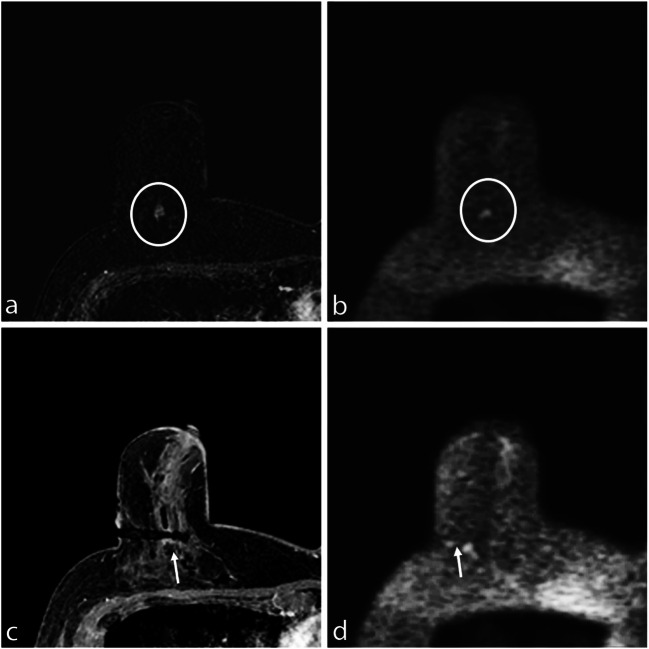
a Subtracted DCE 3D-THRIVE showing a mass lesion in the deep equatorial plane of the right breast of a 58-year-old woman (circle). b DWI (*b* = 1300 s/mm^2^) showing hyperintensity of the enhancing lesion (circle). c T1-WI FS GRE showing the proper position of the biopsy device (arrow); the needle is poorly recognizable at (d) DWI (*b* = 1300 s/mm^2^) due to the deep position of the lesion and local anesthetic injection–related artefacts
